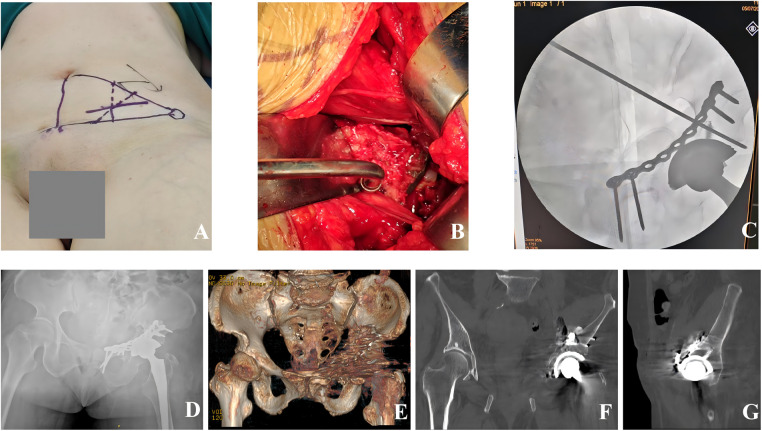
Intraoperative and postoperative images. (A) Patient positioning and incision for the LRAA. (B) Direct visualization of the acetabular fracture and acetabular cup. (C) Fluoroscopic confirmation after reduction and fixation. (D) Postoperative imaging of anteroposterior pelvic radiograph. (E) Postoperative imaging of three-dimensional CT reconstruction. (F) Postoperative imaging of coronal CT image. (G) Postoperative imaging of sagittal CT image.
